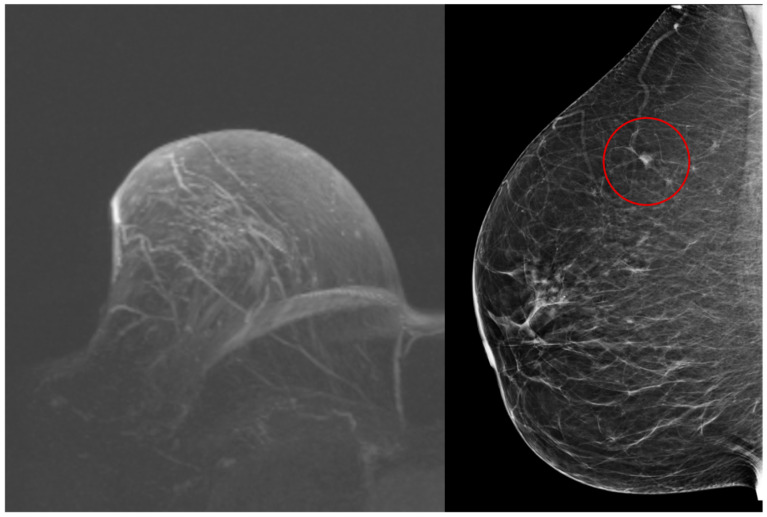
Maximum intensity projection image of breast MRI (left) and mediolateral oblique mammogram (right) in a patient with a new malignancy in the right breast. The mass is visible on mammography (red circle) but not detected on MRI.
